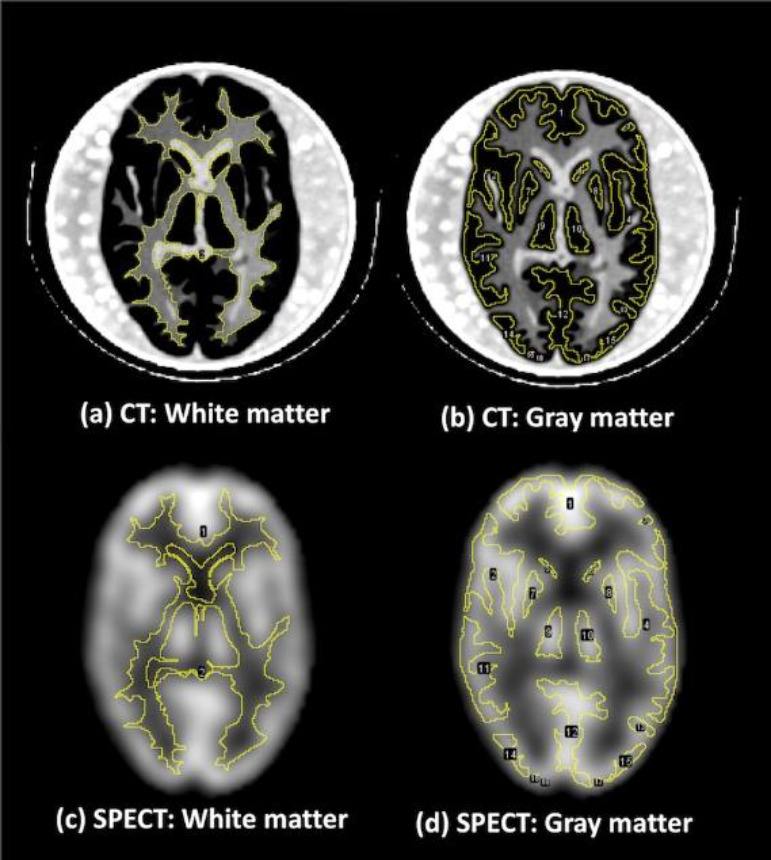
White-matter ROIs on the registered CT image (a) Gray-matter ROIs on the registered CT image (b) White-matter ROIs on the registered SPECT image (c) Gray-matter ROIs on the registered SPECT image (d)
